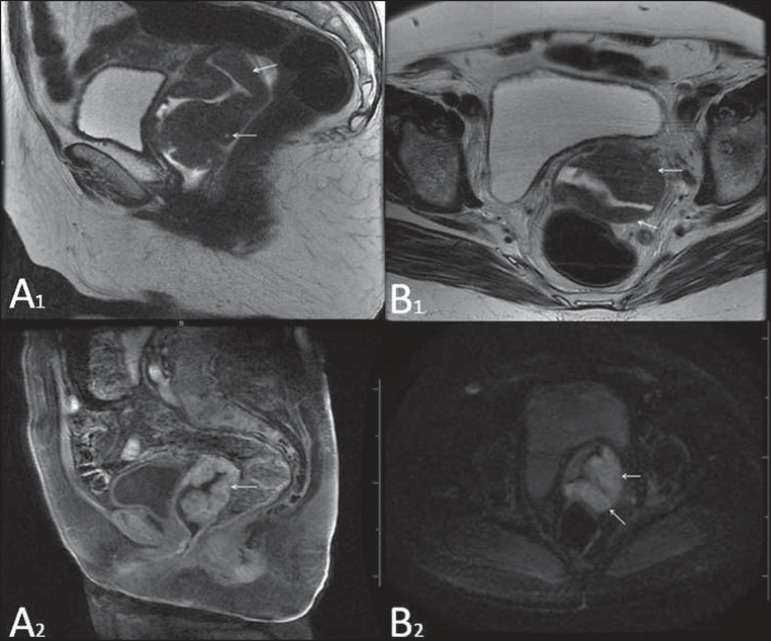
Vaginal melanoma. Sagittal (A1) and axial (B1) MRI
